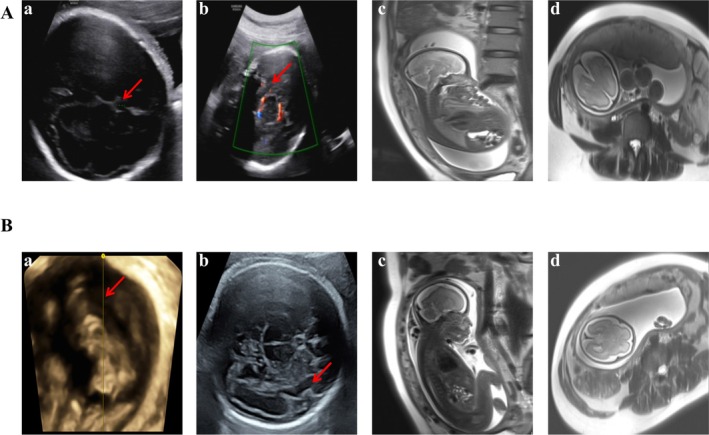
Prenatal ultrasound and MRI findings (A) Fetus II:1. (a, b) Ultrasound at 29^+6^ weeks’ gestation (WG) showed a reduced cavum septi pellucidi (CSP) and agenesis of the corpus callosum (ACC). (c, d) MRI at 30 WG confirmed absent septum pellucidum and corpus callosum, with poor visualization of the anterior horns of the lateral ventricles and optic chiasm. Additional findings included mild hypoplasia of the parieto‐occipital lobes, mild frontal cortical thickening, and an indistinct frontothalamic boundary, suggestive of lobar holoprosencephaly (HPE). (B) Fetus II:2. (a) Ultrasound at 26^+6^ WG indicated partial ACC. (b) Ultrasound at 30^+5^ WG revealed cortical dysplasia with closed Sylvian fissures, simplified gyration, and shallow sulci. (c, d) MRI at 28 WG demonstrated microcephaly, partial ACC, and bilateral cerebral hemispheric abnormalities consistent with a neuronal migration disorder (agyria–pachygyria complex).
